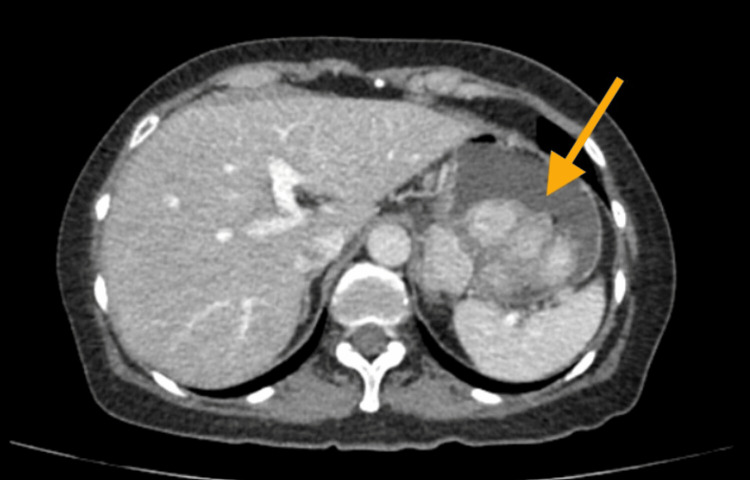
Contrast-enhanced abdominal computed tomography (CT) A mass can be observed in the posteromedial wall of the gastric fundus, multilobulated and enhanced with contrast medium, reaching dimensions of 42 × 52 × 60 mm, indicated by the orange arrow.
